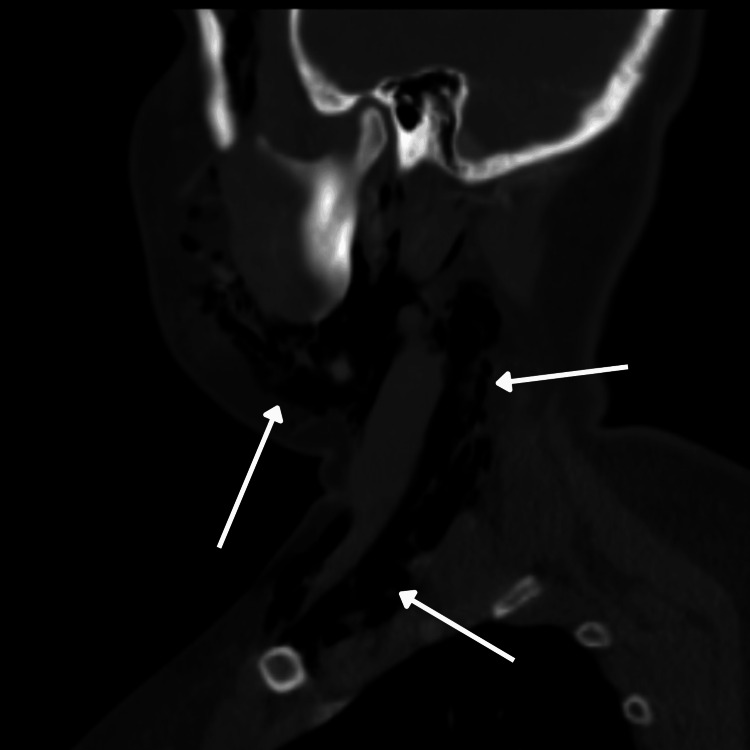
Sagittal contrast-enhanced CT of the neck and thorax A column of gas (arrows) tracks from the submandibular region along the anterior neck into the anterior and superior mediastinum. CT: computed tomography
